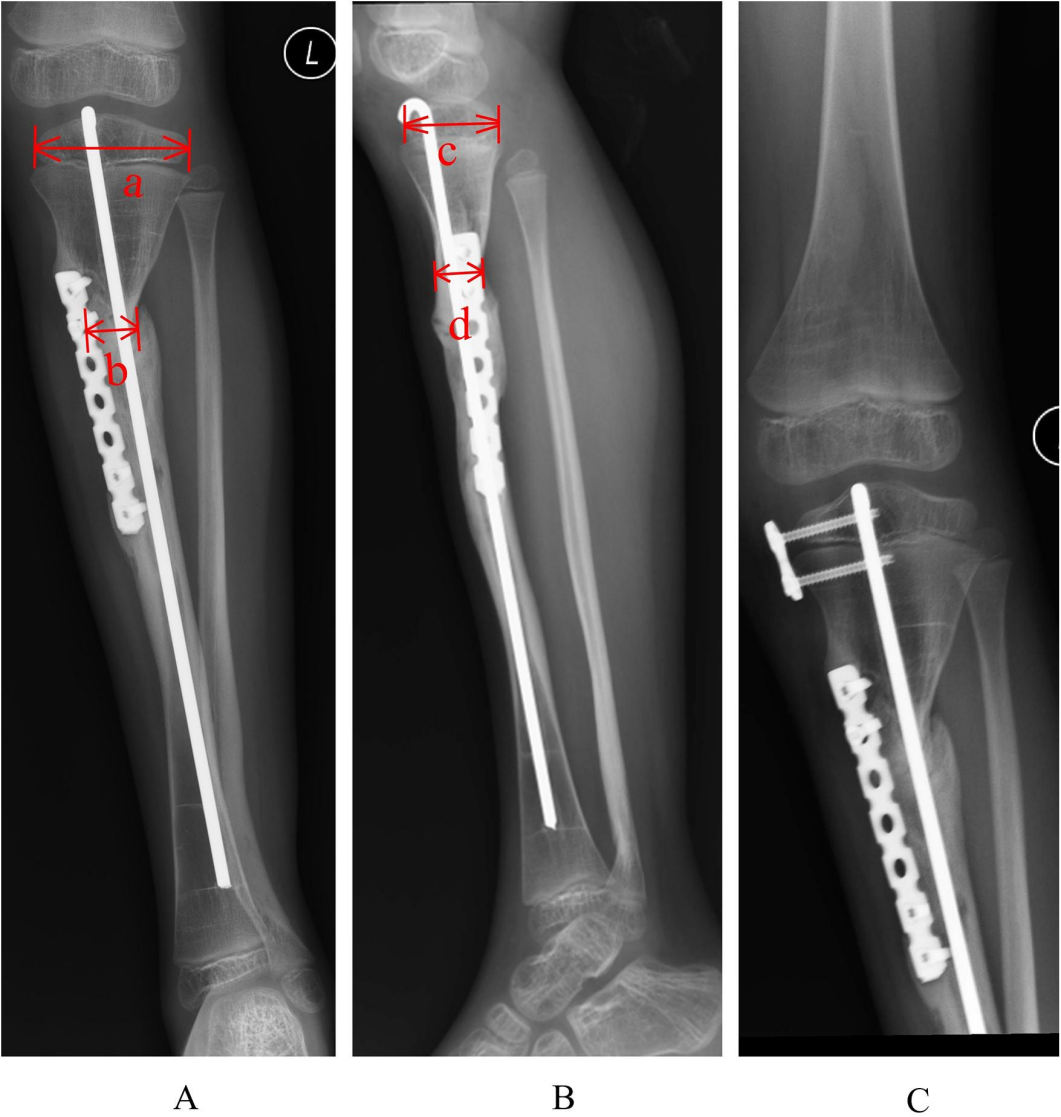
Refracture risk evaluation via anteroposterior and lateral radiographs. The cross-sectional area of the union region is calculated as the product of the narrowest diameter of the pseudarthrosis union on the AP radiograph (A) (denoted as b) and the narrowest diameter on the lateral radiograph (B) (denoted as d). The cross-sectional area of the proximal tibial epiphysis is determined by multiplying the widest diameter of the epiphysis on the AP radiograph (A) (denoted as a) by the widest diameter on the lateral radiograph (B) (denoted as c). The relative cross-sectional area is defined as (b × d)/(a × c).
